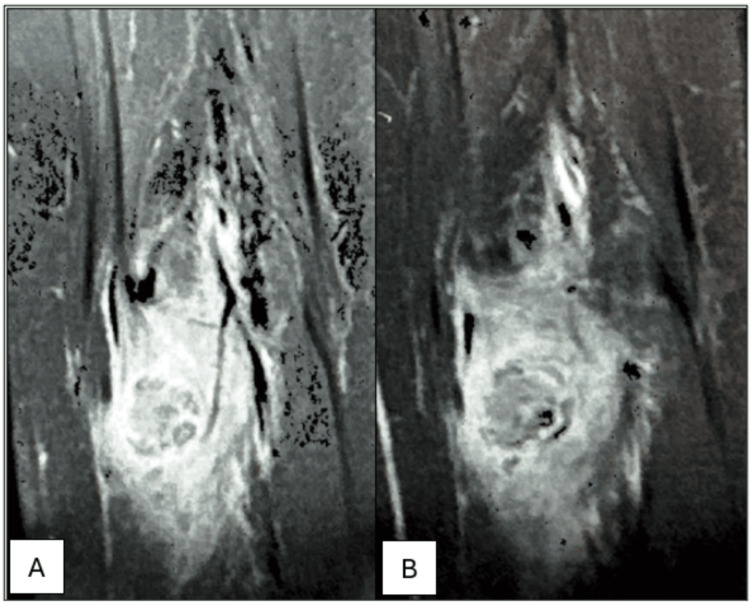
Imaging findings on MRI. A: T1-weighted sequence; B: T2-weighted sequence MRI findings showing a calcified ovoid mass within the soleus muscle, with indistinct margins and heterogeneous signal on both T1 and T2-weighted sequences. A significant inflammatory muscle and bone marrow edema, as well as cortical irregularities with periosteal reaction suggesting an intermediate-stage juxtacortical MO MO: Myositis ossificans
